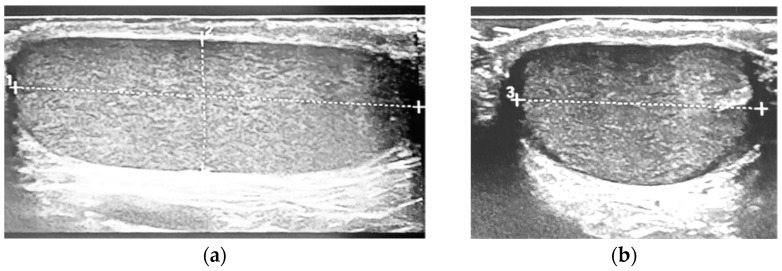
Example of the standardized ultrasound technique used: (a) length (dotted line 1) and depth (dotted line 2) measured on the sagittal view; (b) the probe is then placed per-pendicular and the widthwidth (dotted line 3) is obtained at the broadest point.
